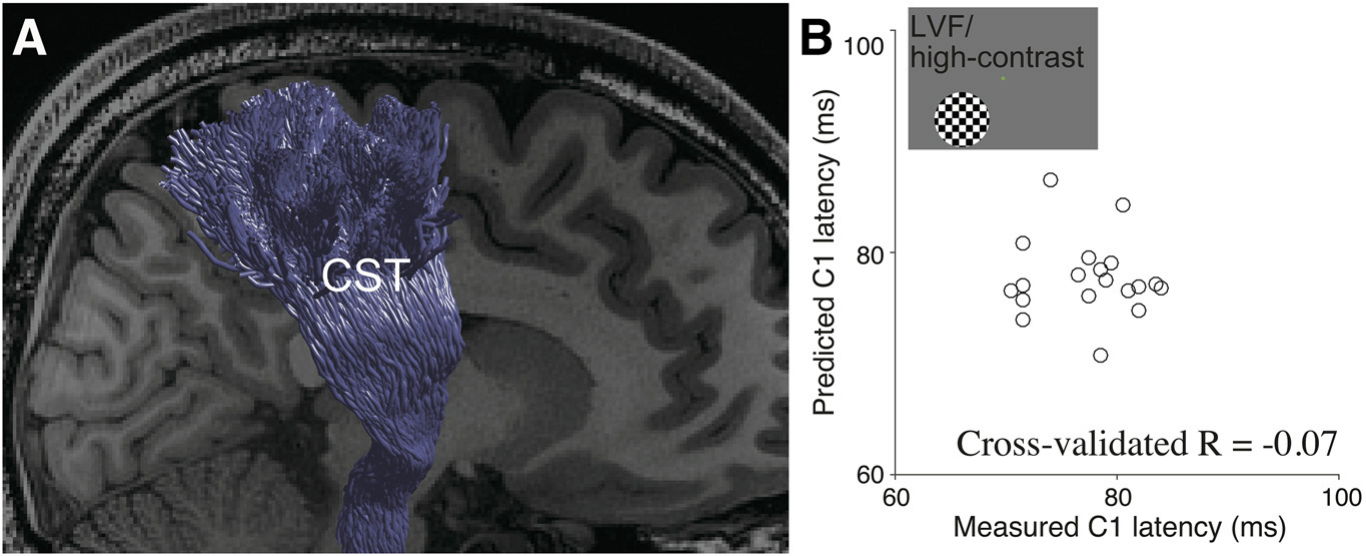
Prediction of C1 peak latency from the corticospinal tract (CST). *A*, The CST in a representative subject (subject 9) identified using tractography in the dMRI dataset overlaid on a sagittal slice of a T1-weighted image. *B*, Comparisons between the measured C1 peak latency (horizontal axis) and the C1 peak latency predicted from the CST (vertical axis) when a high-contrast stimulus was presented at the LVF. The model did not show a significant prediction of C1 peak latency (cross-validated *R* = −0.07, *p *=* *0.60).
